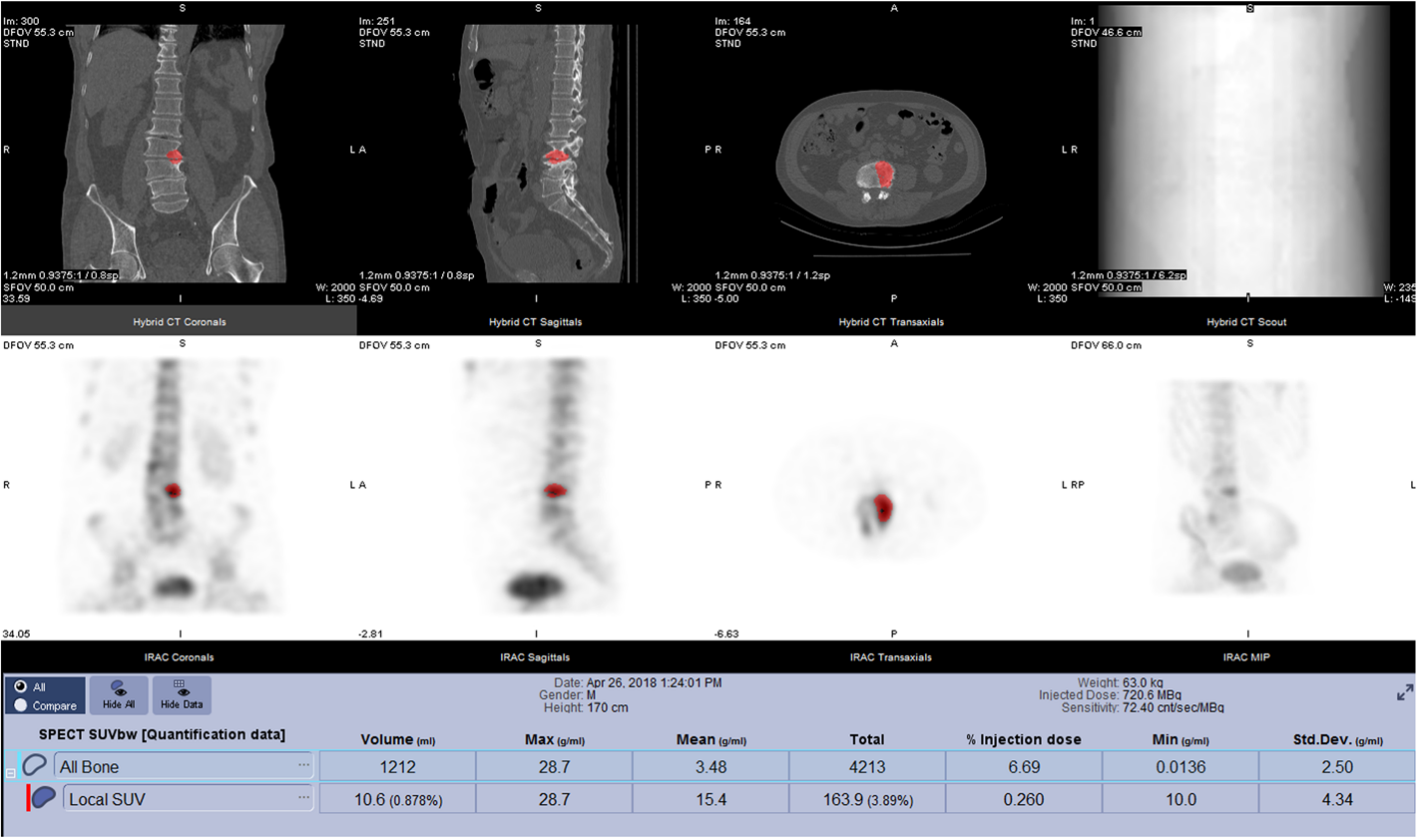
Quantitative bone SPECT of a 69-year-old female patient with a right hip prosthesis who is experiencing bone pain. Data show degenerative changes at the L3 and L4 endplates and elevated SUV
